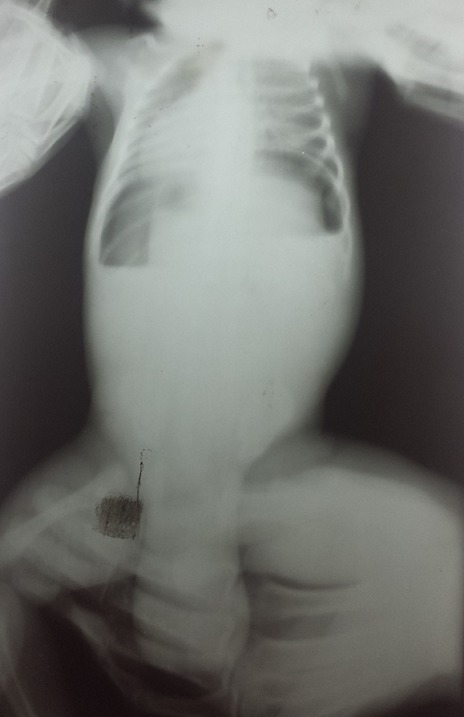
Radiographie abdomen sans préparation qui montre un large pneumopéritoine
